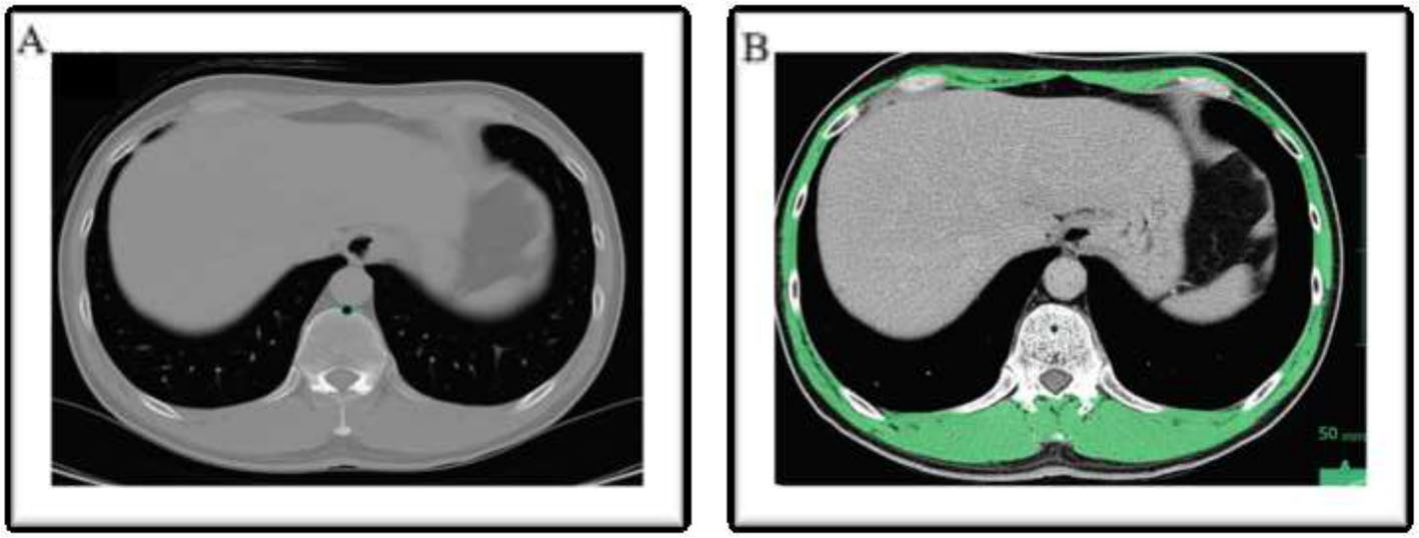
a The original CT image at Th12 transferred in the DICOM format. b The tissue HU threshold was set from − 29 to + 150 HU for skeletal muscle. The skeletal muscle was calculated by excluding the abdominal viscera and Th12
